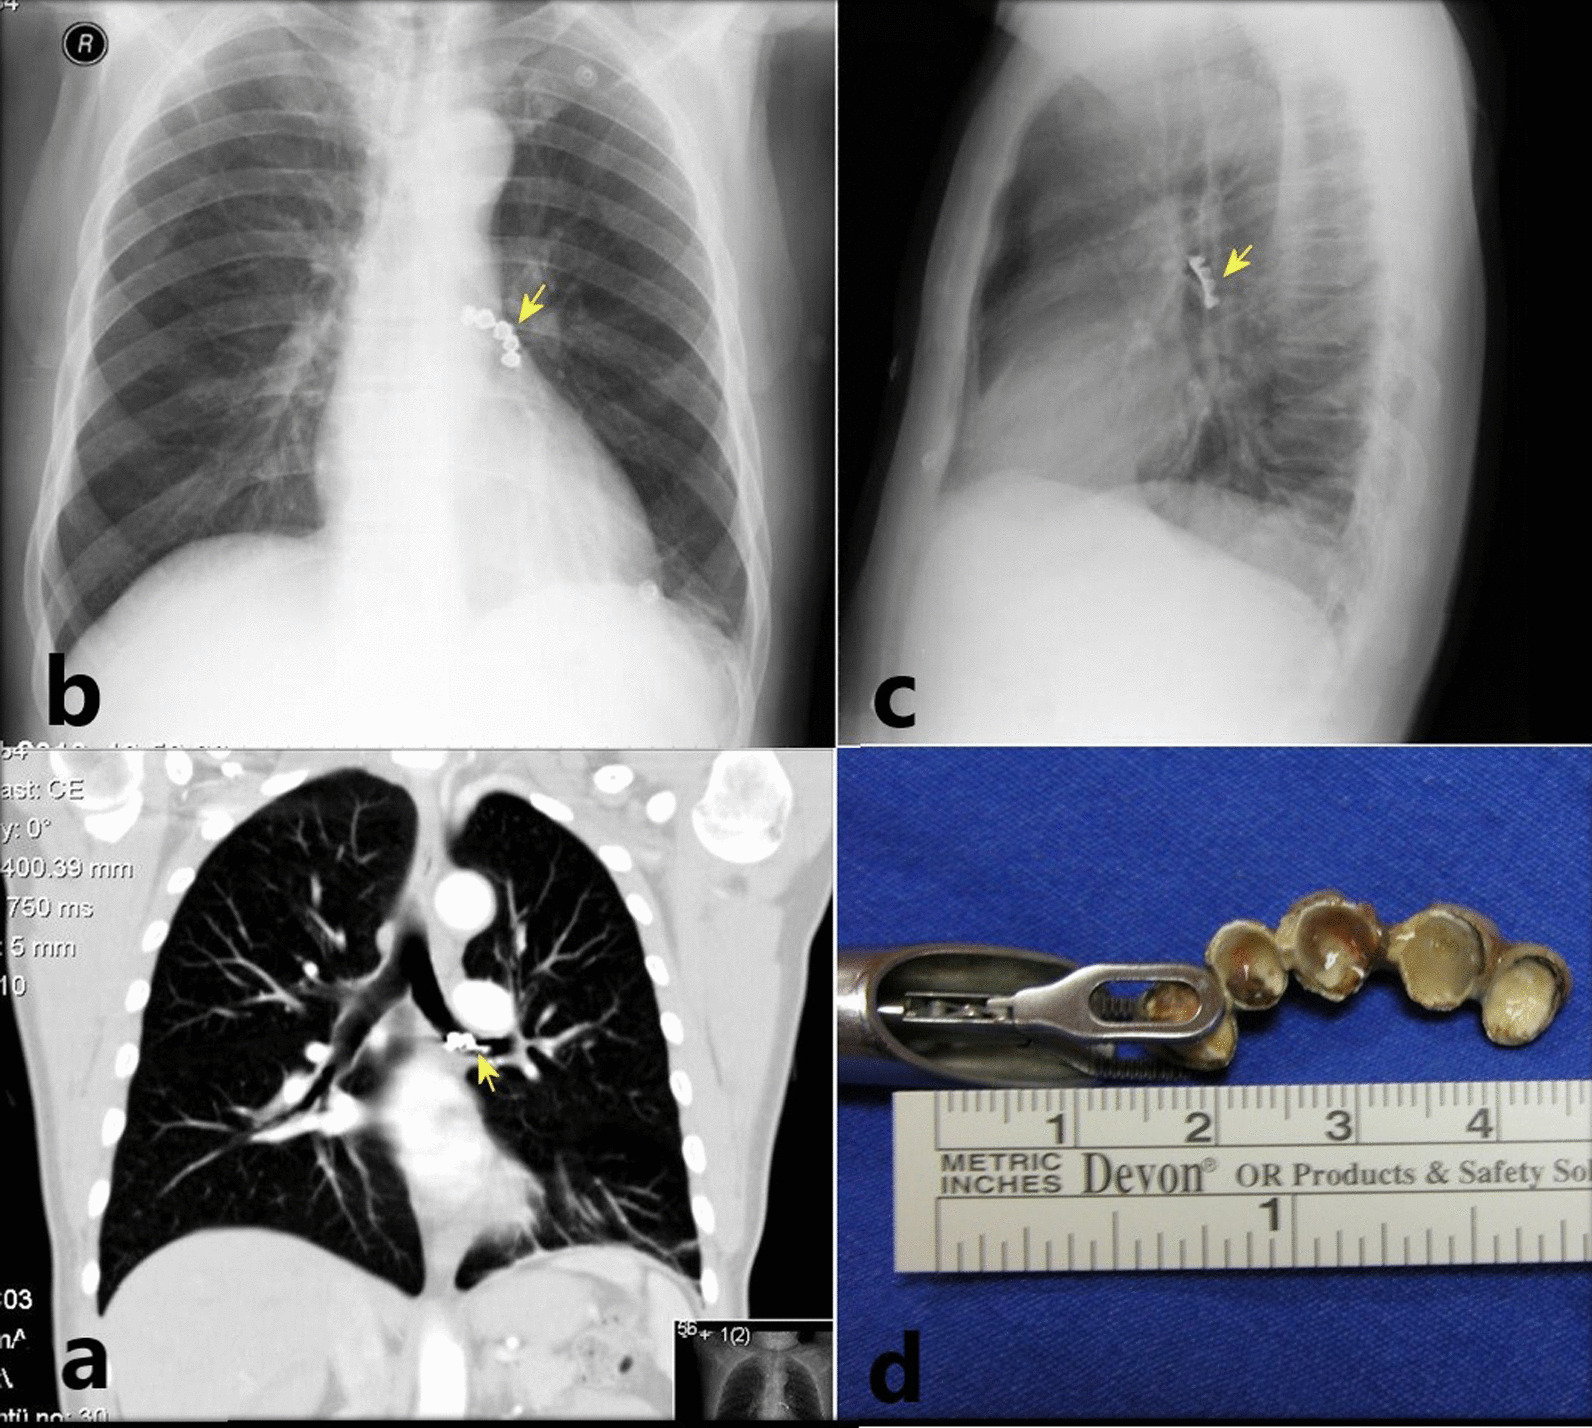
Lower jaw bridge prosthesis. a Coronal section of computed tomography of the thorax; yellow arrow shows molar crown in the left main lobe bronchus. b, c Posterior-Anterior (PA) and lateral chest X-ray; black and red arrow shows dental screw. d Rigid bronchoscope forceps and bridge prosthesis
